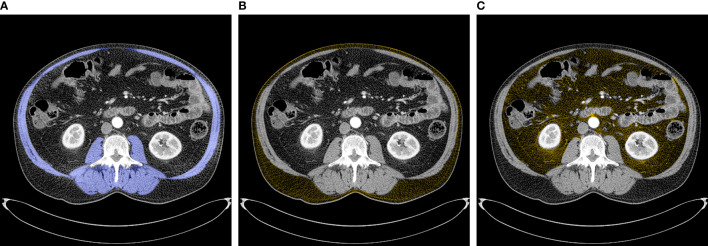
Abdominal CT images at the third lumbar vertebrae used to quantify body composition variables. (A) Skeletal muscles (blue) (B) Subcutaneous adipose tissue (yellow) (C) Visceral adipose tissue (yellow).
